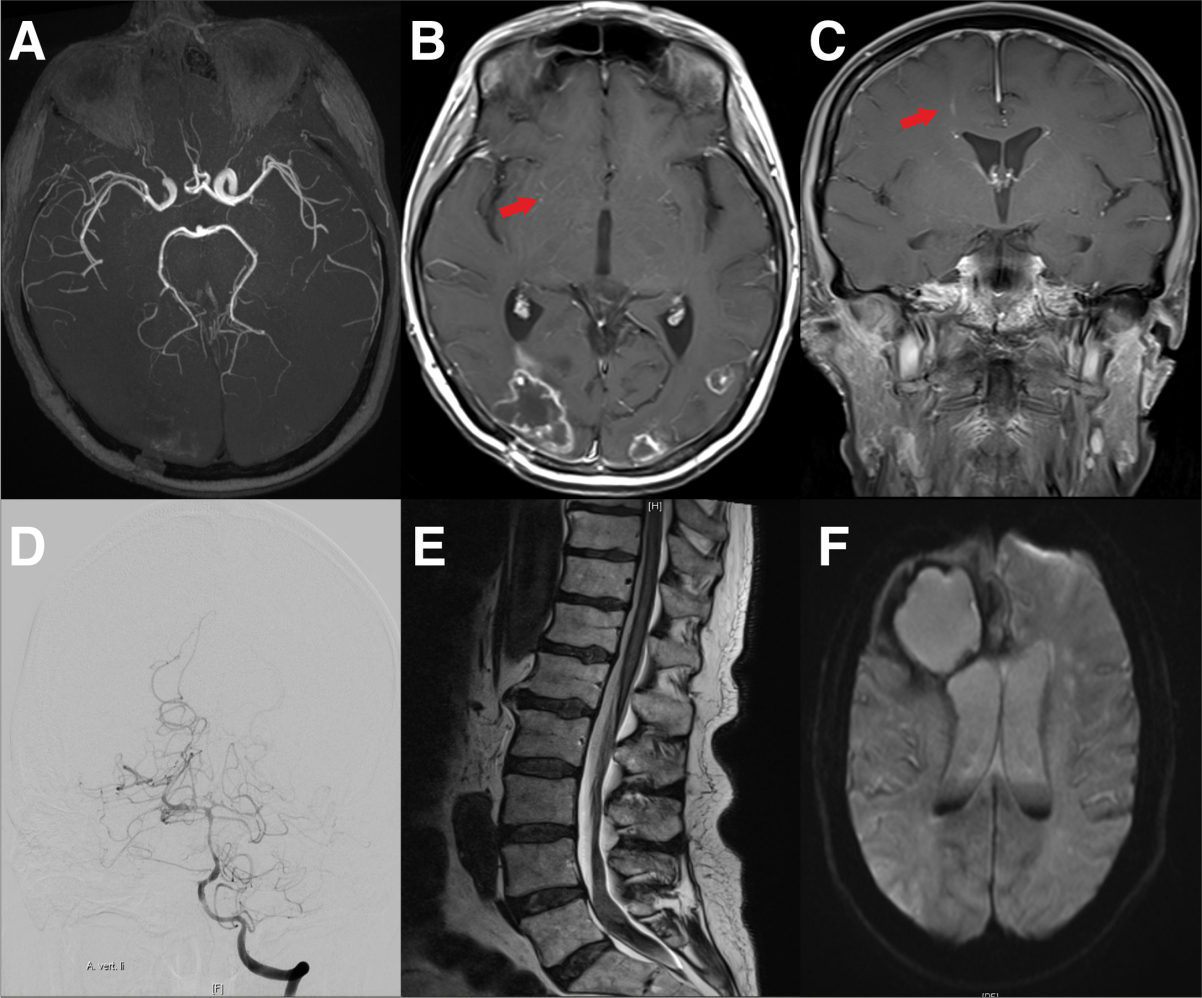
Cranial MRI of a 44-year-old female patient with NSCLC and pembrolizumab therapy. (A) Shows vascular imaging with no evidence of vasculitis-type changes in the large cerebral vessels. (B) Transversal gadolinium enhanced T1 weighted MRI with the remains of the occipitally located brain metastasis, as well as periventricular contrast medium accumulations suspicious for vasculitis (arrow). (C) A frontal gadolinium enhanced T1 weighted MR of the same patient. Again, the arrow indicates suspicious contrast agent accumulations. (D–F) Cranial MRI Scans and vertebral column MRI of a 72-year-old patient with melanoma. The Patient had been treated with nivolumab for 15 months. The patient then developed a headache and paraparesis. Cerebral angiography showed caliber changes of the left middle cerebral artery and the basilar artery. Due to vasculitis, the patient developed prolonged bleeding with siderosis-associated myelopathy. (D) Cerebral angiography with caliber changes of the cerebral vessels. (E) Cranial MRI of the lower spinal cord with bleeding in the caudal region. (F) Cranial MRI with bleeding of the metastasis and blood in the liquor system.
